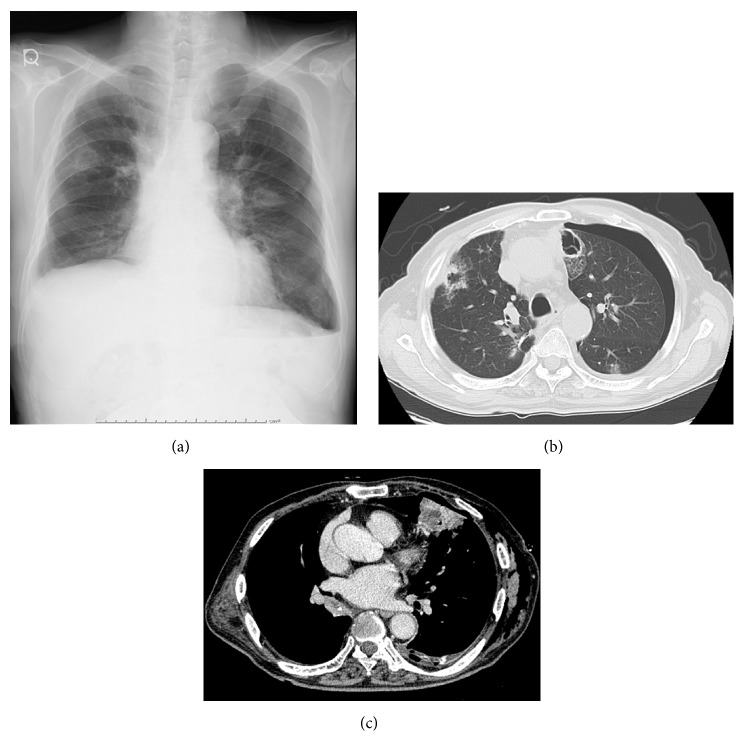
(a) A chest X-ray showed a left pneumothorax and multiple metastases in the bilateral lung. (b) A CT scan of the chest revealed that some nodules with cavities were located in the edge of the lung. (c) A contrast enhanced CT scan of the chest revealed a cavity, culprit lesion of hemoptysis, 25 mm in size, in the left upper lobe.
